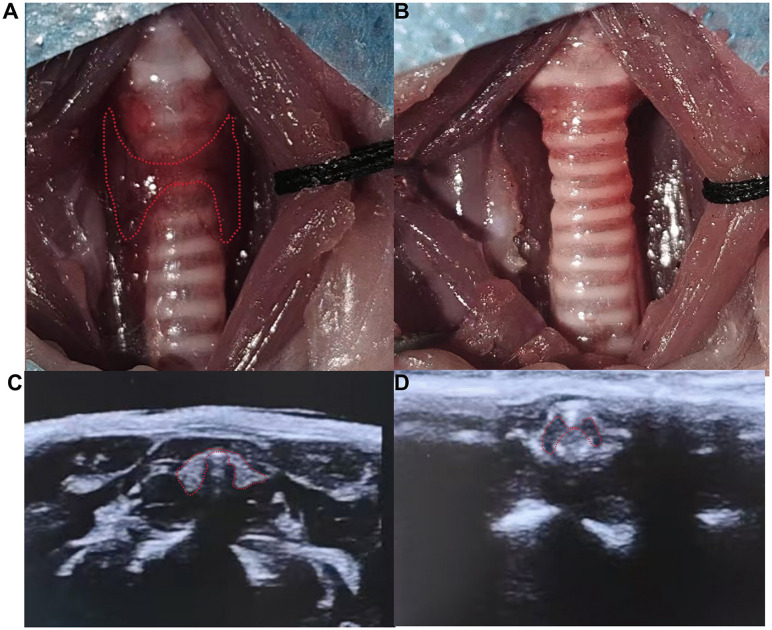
Preoperative and postoperative thyroid visual field exposure and ultrasound exploration. (A) Intraoperative exposure of thyroid tissue. (B) Visual field performance after total thyroidectomy. (C) Preoperative thyroid ultrasound imaging. (D) Ultrasound imaging after total thyroidectomy.
